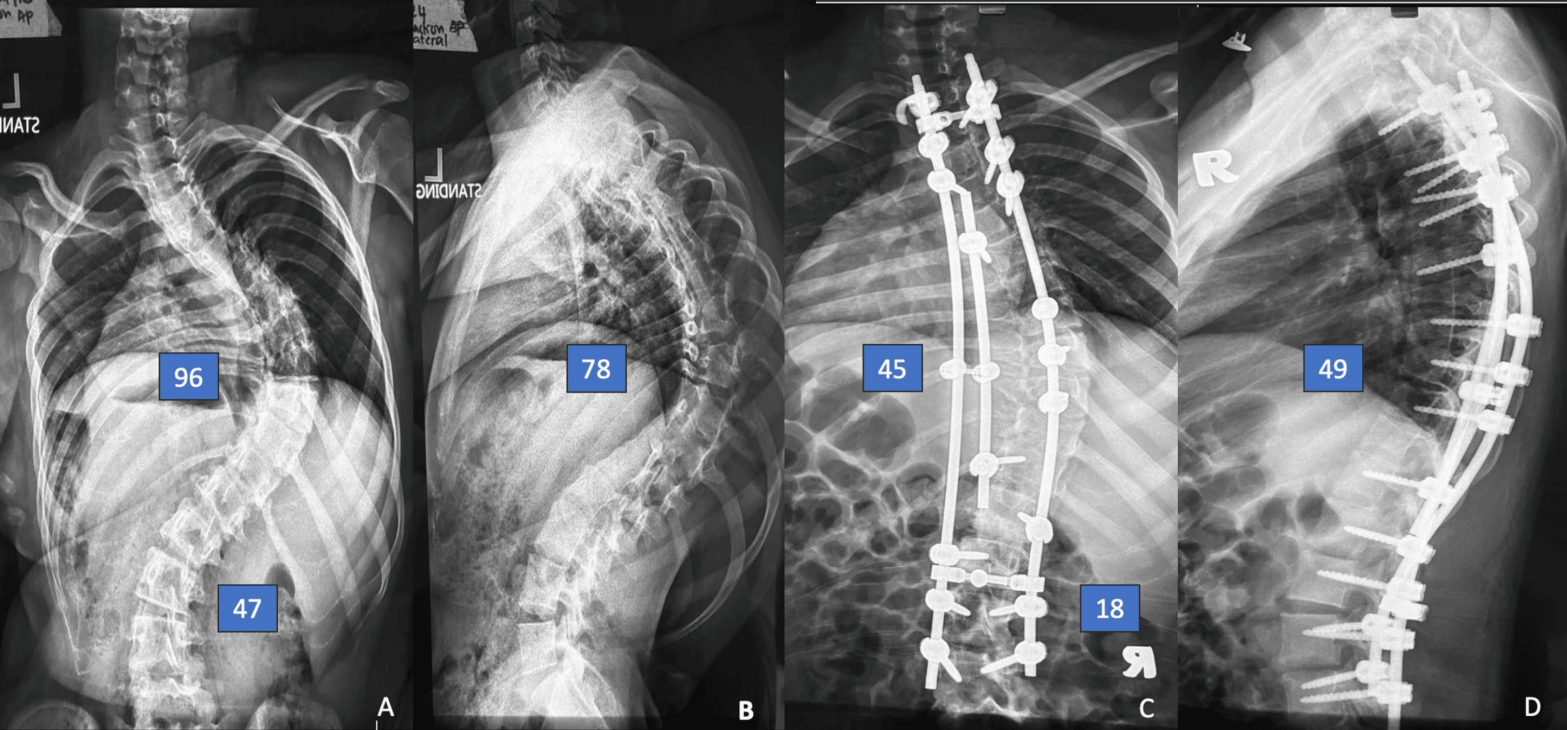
Whole spine radiograph of patient number 4. The pre-operative main thoracic (MT) Cobb angle of 96°, thoracolumbar/lumbar (TL/L) 47° (A) and thoracic kyphosis 78° (B). She underwent posterior instrumentation and fusion using triple-rod technique from T2-L4. The post-operative x-rays show coronal curve correction of MT to 45°, TL/L to 18° (C), and kyphosis correction to 49° (D). The details are provided in Table 2.
